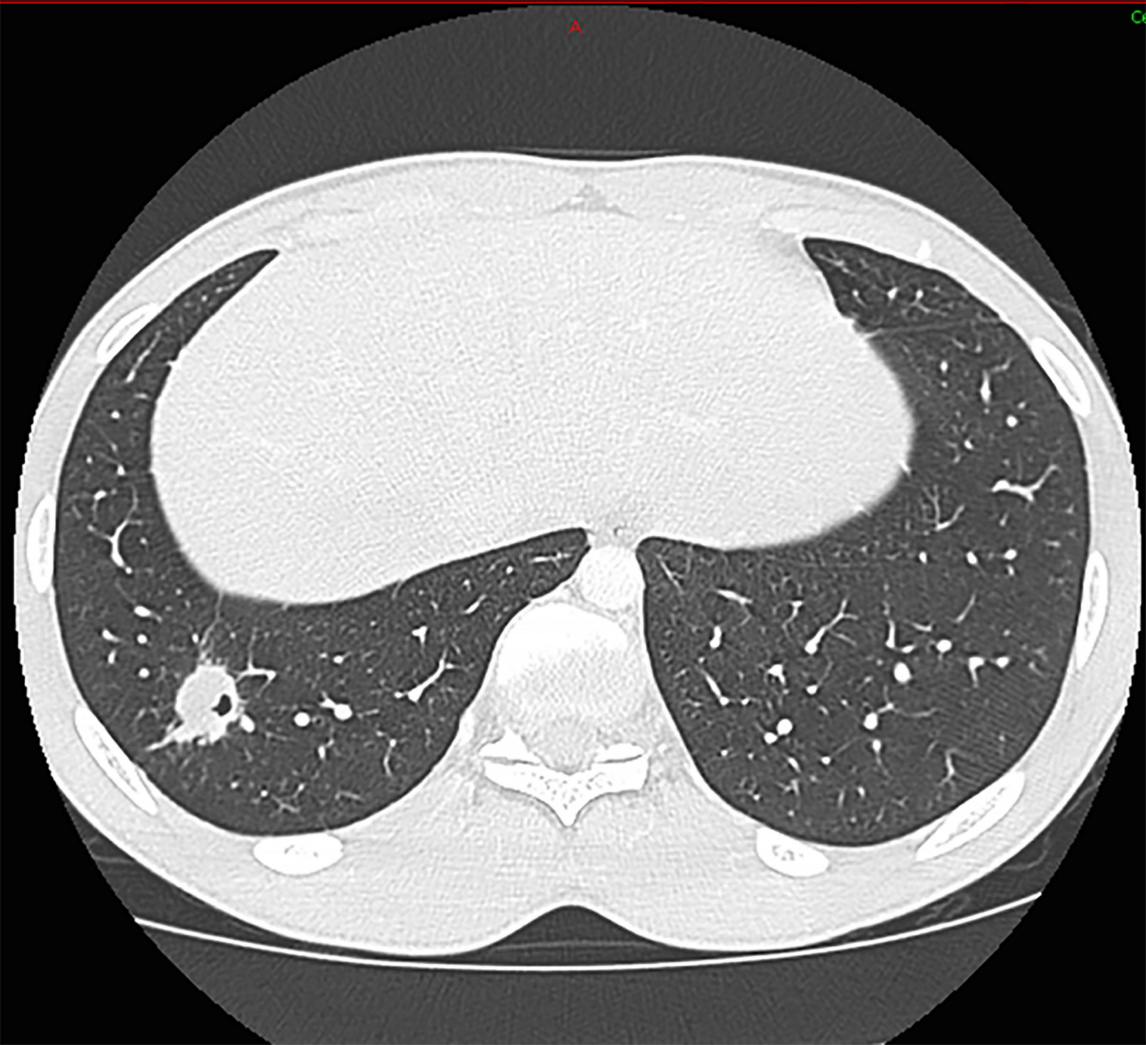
Pulmonary Computed Tomography–scan with pulmonary nodule of the right basad pyramid excavated.
